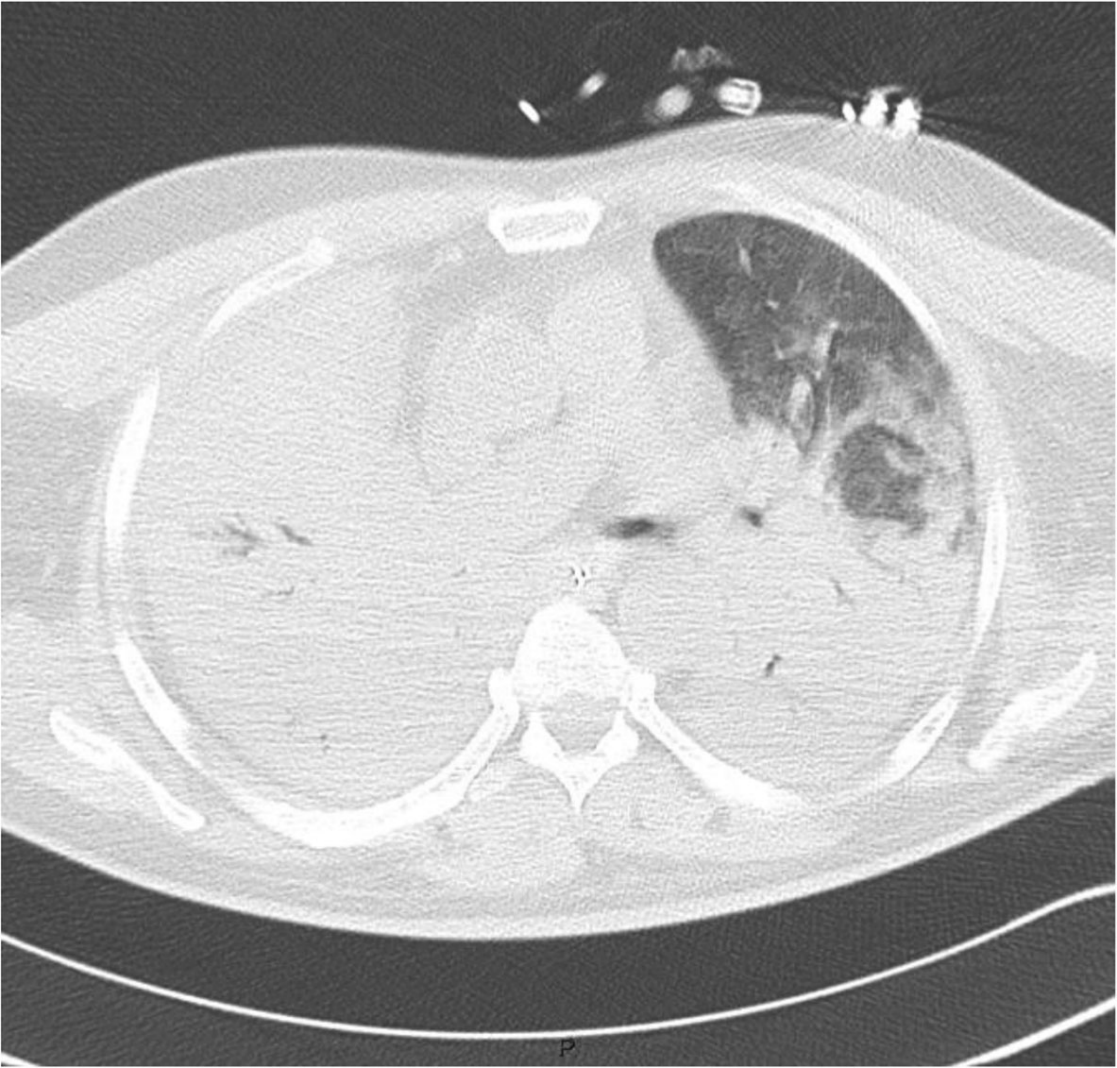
Chest computed tomography angiography without vascular abnormalities. A complete condensation of the right lung and three-quarter condensation of the left lung may be observed
